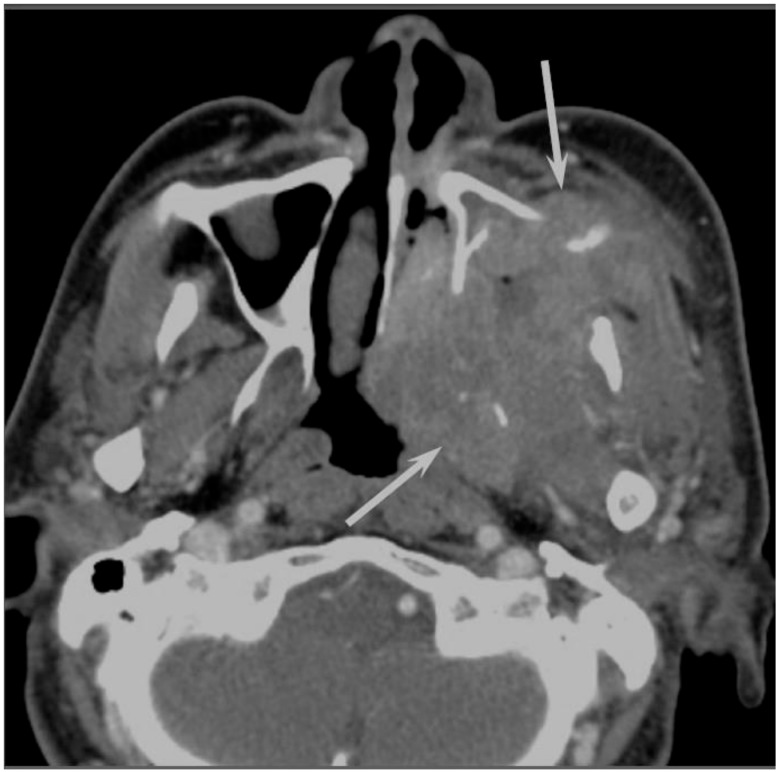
Squamous cell carcinoma of the left maxillary sinus. Contrast-enhanced CT image showing an ill-demarcated, heterogeneously enhanced bulky mass with extensive bony destruction (arrows).
